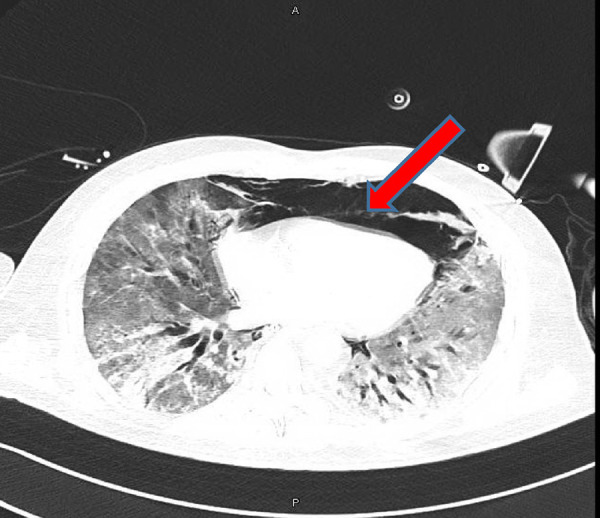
Computed tomography scan showing tension pneumomediastinum
